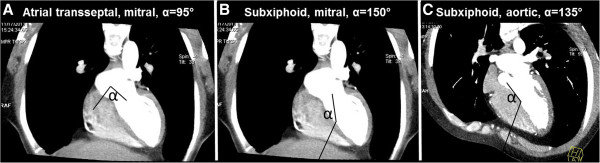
Theoretical trajectories from human CTA. (A) “Conventional” atrial transseptal trajectory to the mitral valve has entry angle (α) = 95°. (B) Subxiphoid trajectory to the mitral valve across the RV and interventricular septum, α = 150°. (C) Subxiphoid trajectory to the aortic valve across the RV and interventricular septum, α = 130°. These also depict the distances reported in Table 2.
